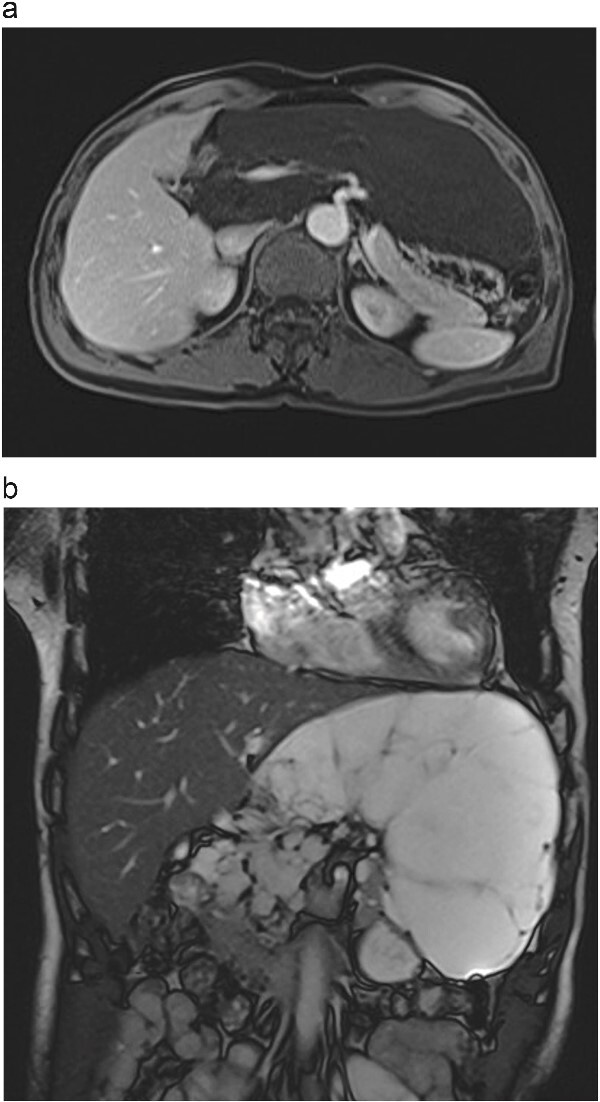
(a) Abdominal contrast-enhanced scan in the arterial phase shows the tumour compressing the portal vein, common hepatic artery, and celiac trunk. (b) T2-weighted MRI image shows the tumour with high signal intensity and no solid components.
